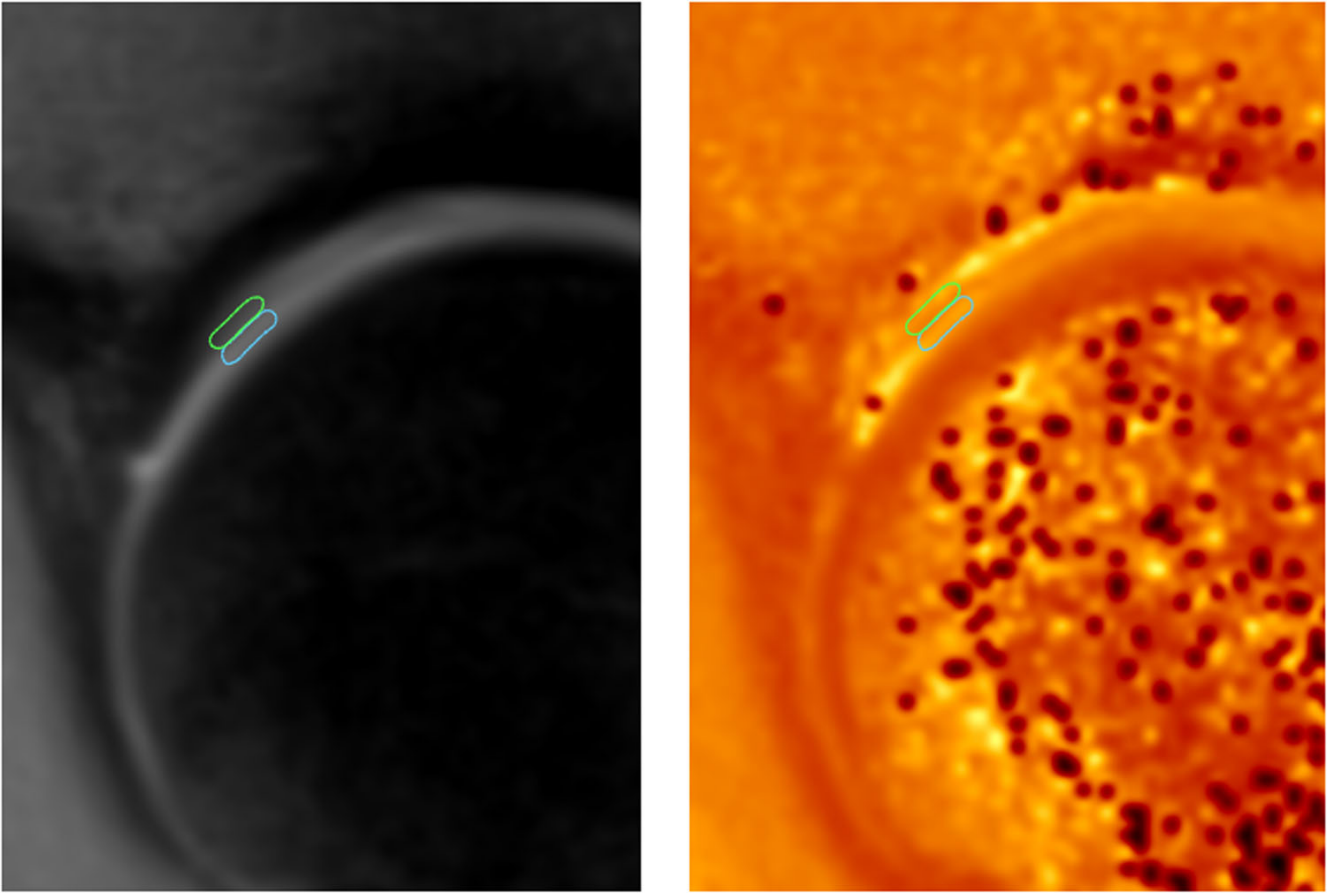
Region of interest (ROI) definition at the chondrolabral junction for delayed gadolinium‐enhanced MRI of cartilage (dGEMRIC): The dGEMRIC analysis was conducted by defining acetabular and femoral head cartilage ROIs at the chondrolabral junction, reaching approximately 3–6 mm toward the acetabular fossa in each hip, on three midsagittal plane slices. MRI, magnetic resonance imaging.
